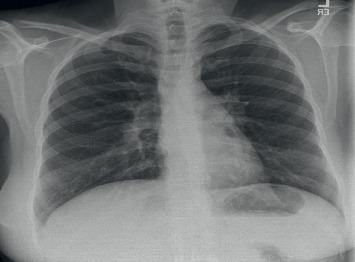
Chest X-ray showing no abnormality or masses.
